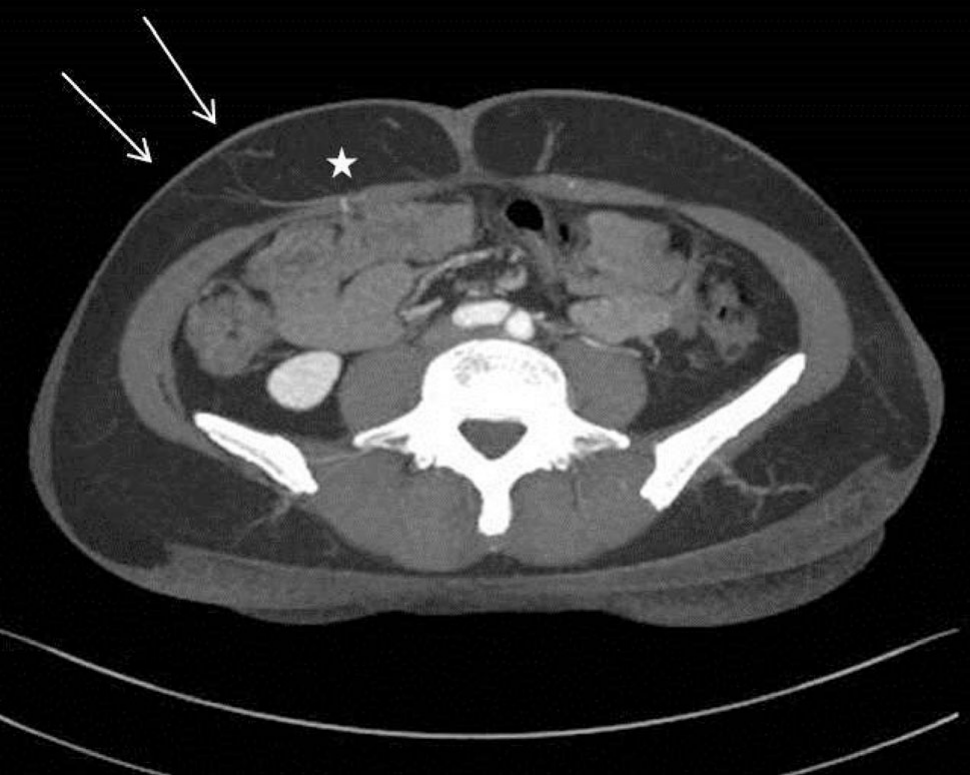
MDCT picture before DIEP breast reconstruction showing differences between actual position of perforator and Doppler signal. *Star* indicates real position of perforator piercing the fascia. *White arrow* indicates the position of audible preoperative Doppler signal
